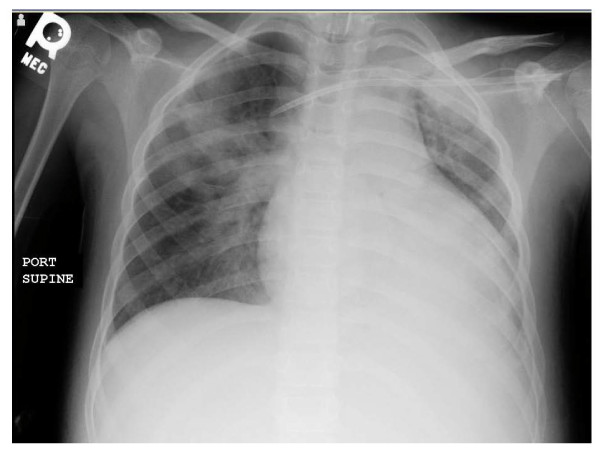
Chest X-ray showing pulmonary hemorrhage. Diffuse alveolar opacification is apparent, particularly in the right upper lobe. Bronchoalveolar lavage revealed fresh blood and hemosiderin laden macrophages.
